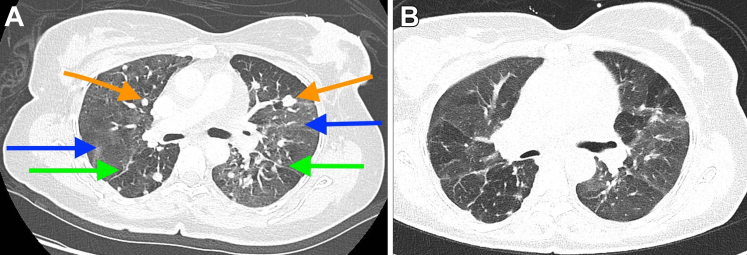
A, CT chest scan showing bilateral ground-glass opacities (blue arrows), pulmonary nodules (orange arrows), and septal thickening (green arrows). B, Follow-up CT scan with resolution of the nodules.
